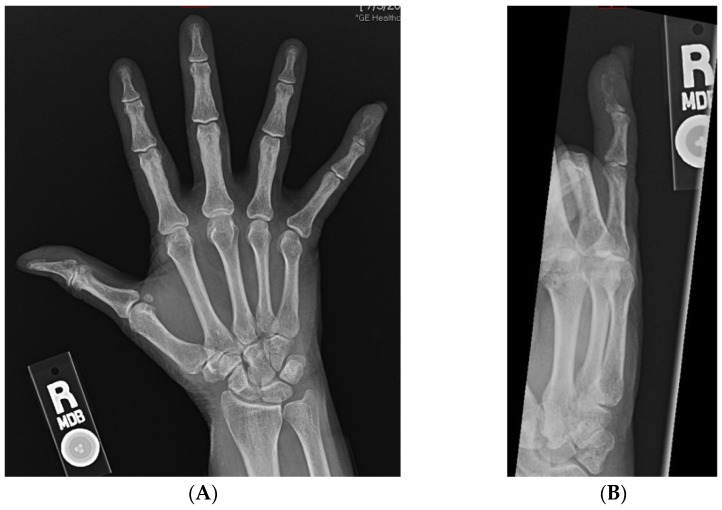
(A,B): AP and oblique radiographs of the right hand demonstrating lytic destruction and pathologic fracture of the distal phalanx of the small finger.
